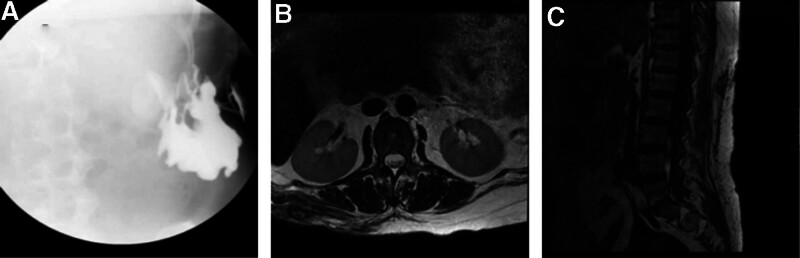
(A) X-ray imaging revealed an irregular sinus tract in the right lumbar region, measuring approximately 10.0 × 9.0 cm. (B, C) MRI showed a patchy, heterogeneous signal at the L2 level, with a maximum cross-sectional area of about 7.8 × 6.3 cm. MRI = magnetic resonance imaging.
